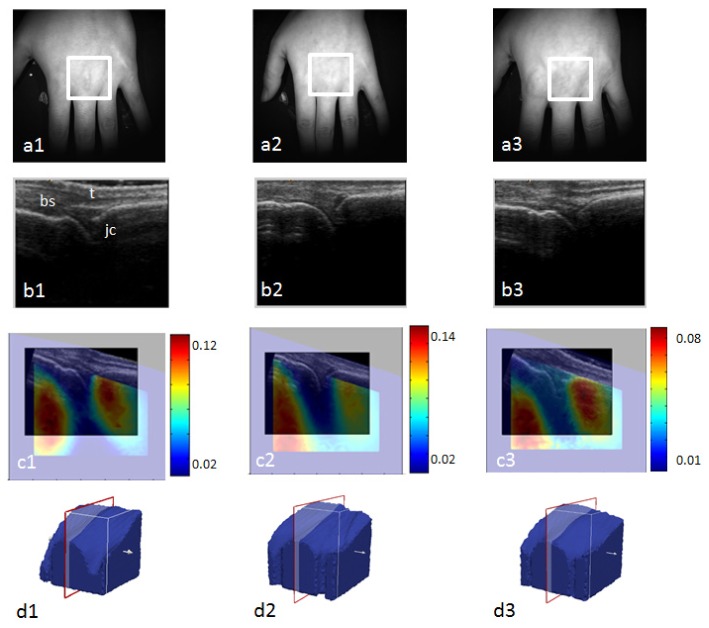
Reconstructed images of the HbT concentration (unit: milli molar) in the third MCP joint from three volunteers, overlapped on the ultrasound image co acquired on the same location. a1) – a3) the bright field image for each volunteer. the region used for DOT reconstruction as indicated by the white frame. b1) – b3) the co acquisition of ultrasound image. bs: bone surface; t: tendon; jc: joint cavity. c1) – c3) registered DOT images and ultrasound images. d1) – d3) the position of the DOT image and ultrasound image slice position for each volunteer.
